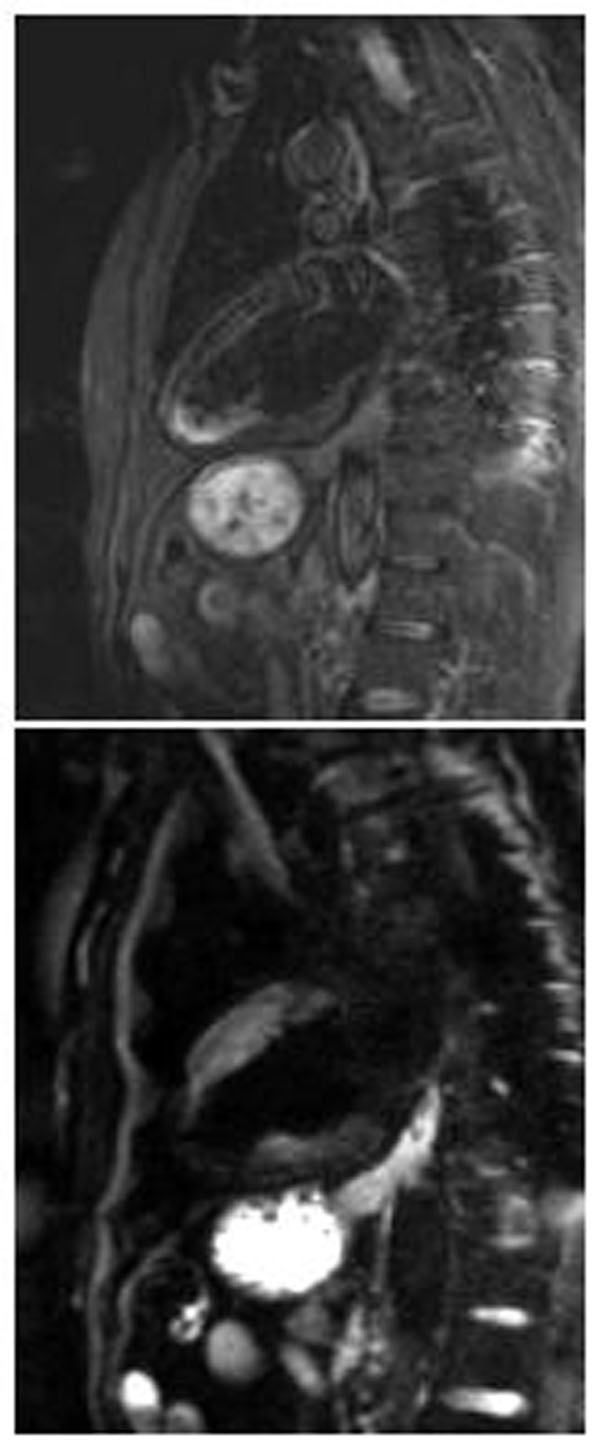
Myocardial oedema in 2 chamber view evaluated by A) STIR T2-weighted image; B) DW EPI sequence
